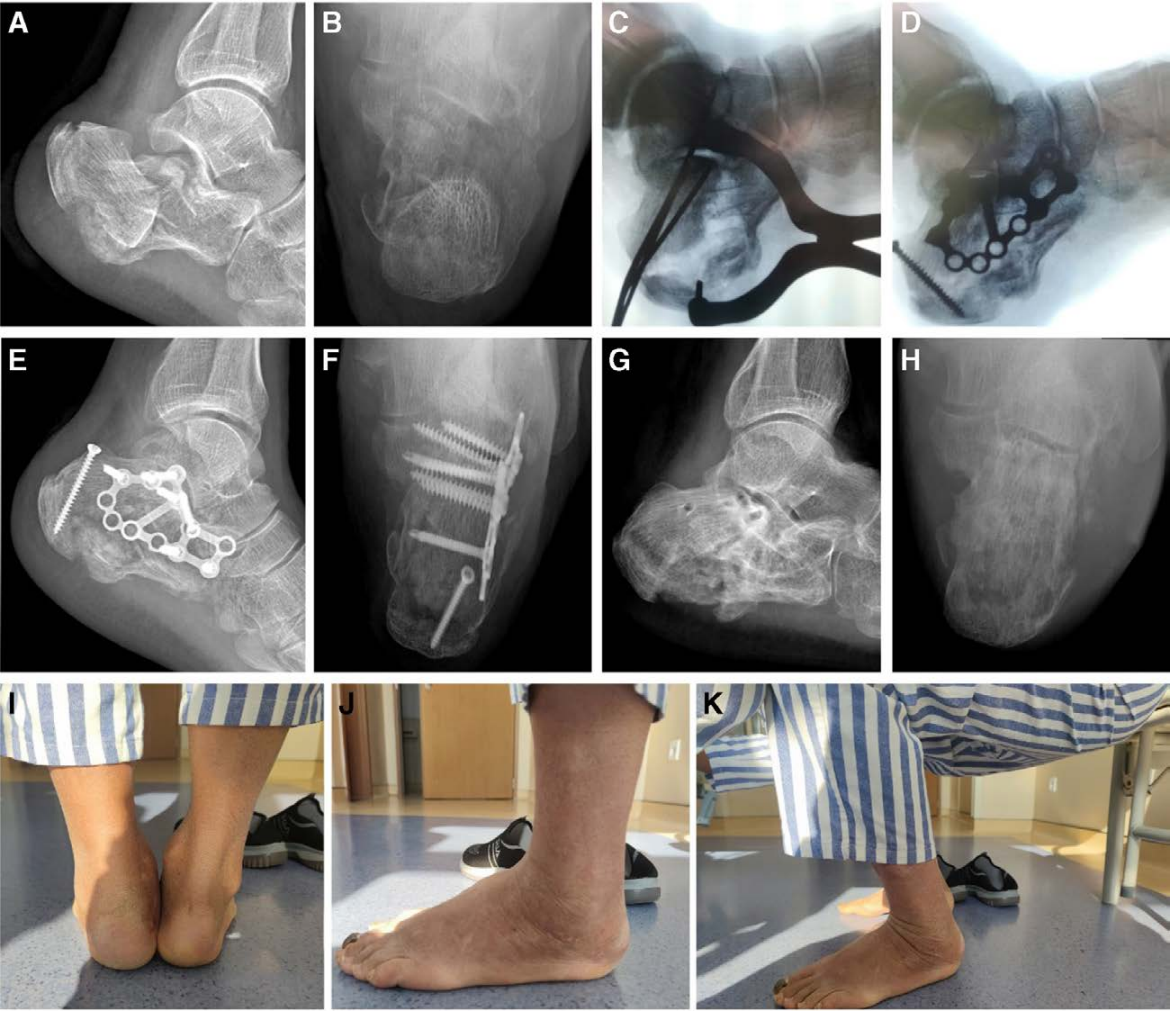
A 61-year-old male patient had a car accident. (A and B) Preoperative radiograph showing left calcaneal fracture (Sanders type ⅢAC, Tough type) with unnormal Böhler angle (–65°), height (91.8 mm), length (92.8 mm), and width (65.4 mm). (C and D) The protocol of Steinmann pin retractor-assisted reduction with circular plate fixation via the sinus tarsi approach was used, and the tuberosity fragment was fixed with a cancellous screw. (E and F) The postoperative radiograph showing the Böhler angle (32°), height (91.1 mm), length (89 mm), and width (39.9 mm) are restored. (G and H) At the last follow-up, the radiological parameters were well maintained. (I, J and K) The patient had a normal alignment of the calcaneus and stable plantigrade foot, and the AOFAS score was 96. AOFAS = American Orthopedic Foot and Ankle Society.
